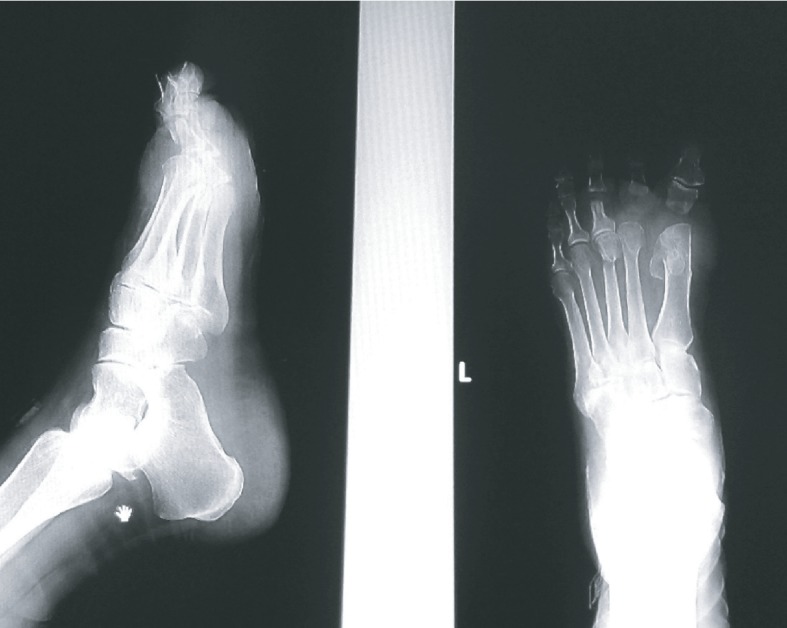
X-ray of the patient's left foot at the first postoperative day.
